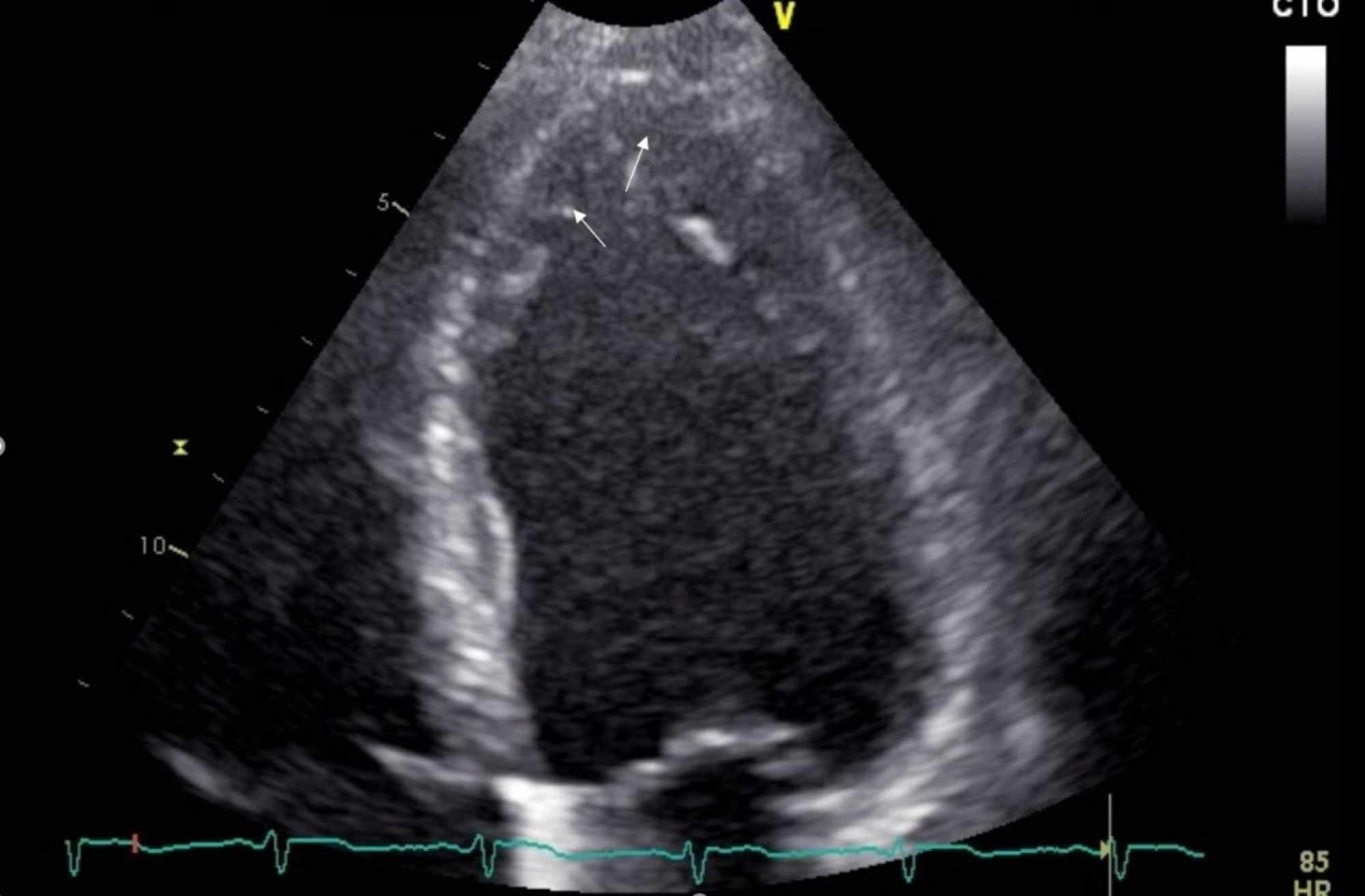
TTE image showing trabeculations (arrows) TTE: Transthoracic echocardiogram
